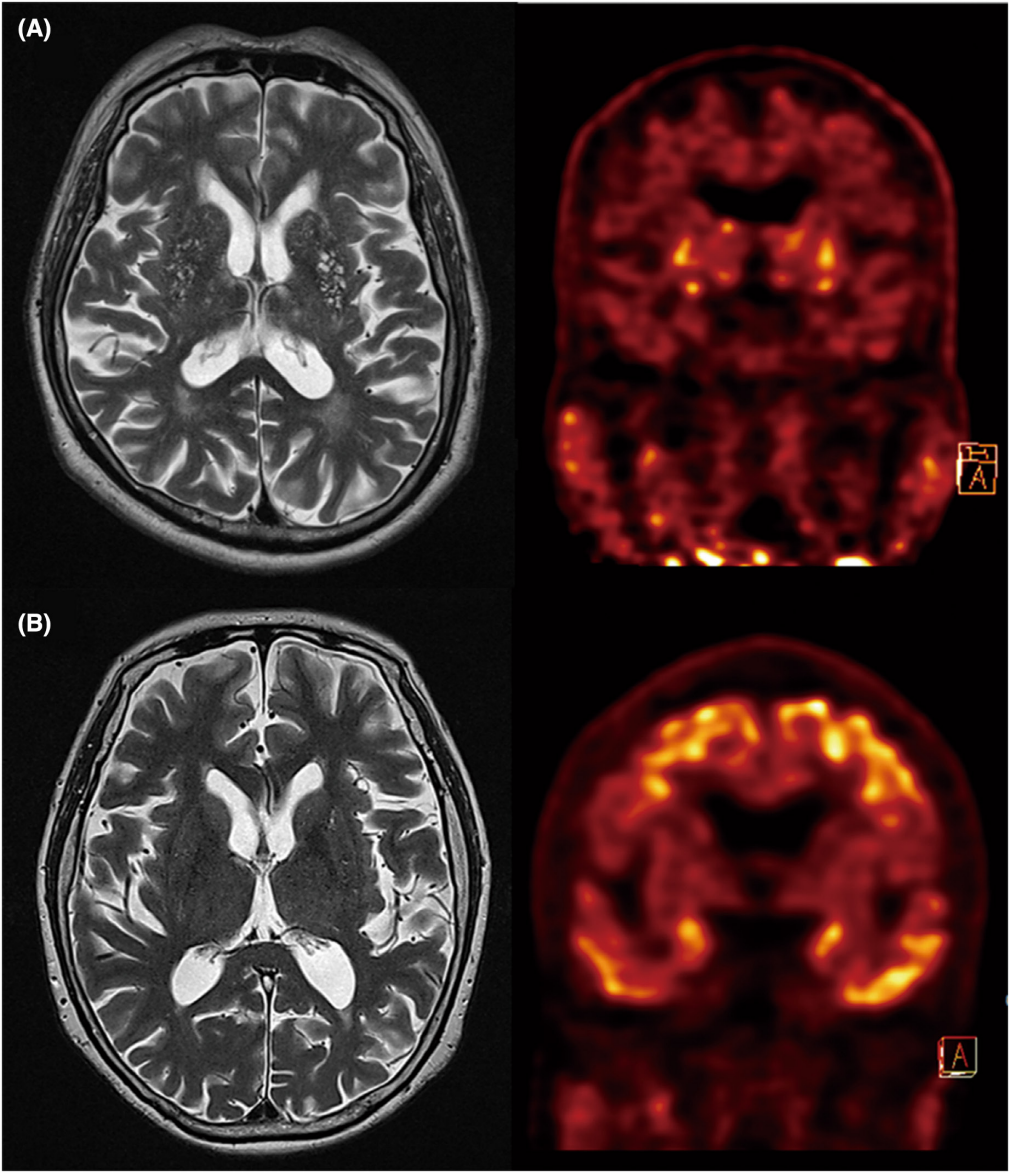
T2‐weighted MR images and tau PET images in two representative cases. (A) a 79‐year‐old man with high‐degree of BG‐EPVS and low brain tau deposition (0.1 SUVR). (B) a 68‐year‐old woman with low degree of BG‐EPVS and high brain tau deposition (4.25 SUVR). BG‐EPVS, enlarged perivascular spaces in the basal ganglia; SUVR, standardized uptake value ratio.
